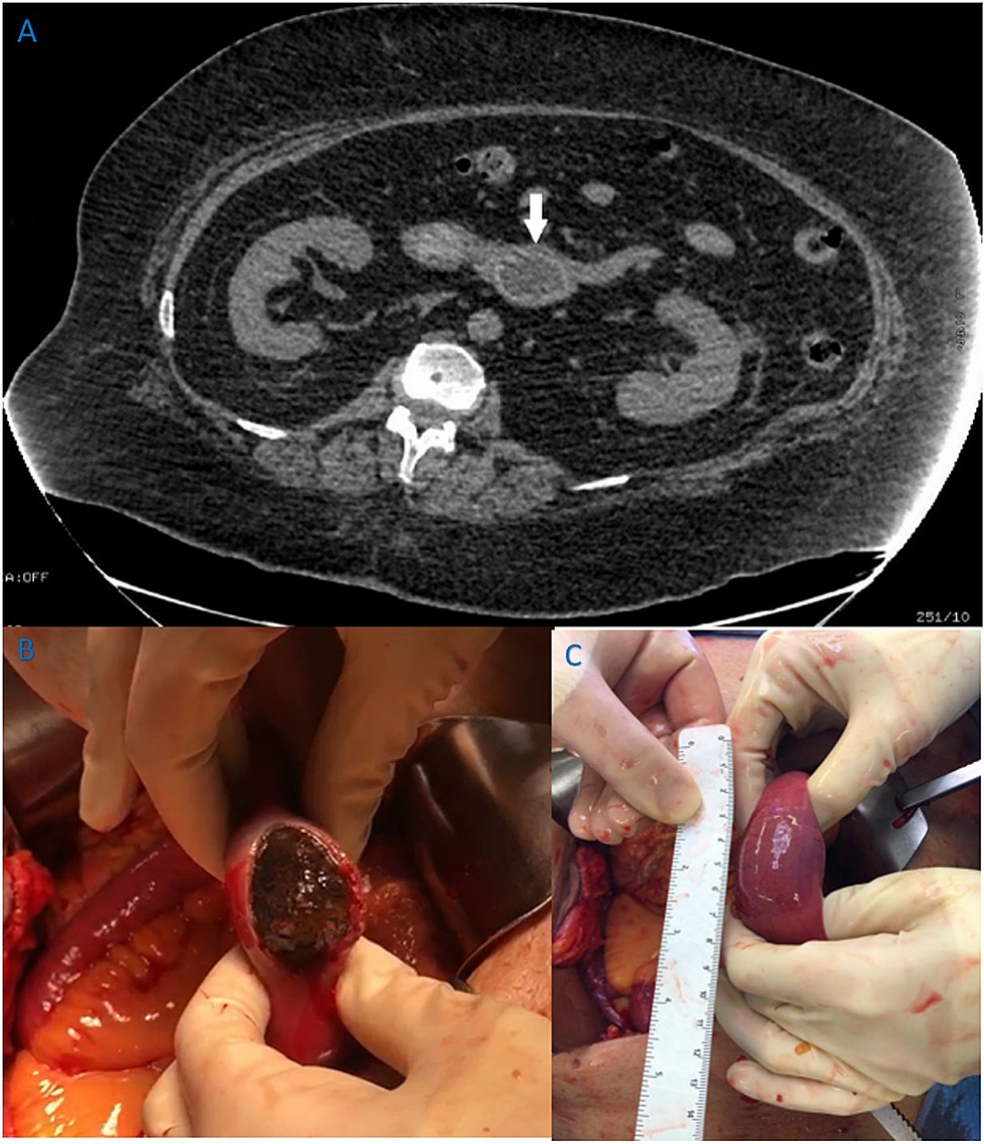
Gallstone ileus. (A) CT scan showing gallstone obstructing ileum (white arrow). (B) Obstructive gallstone in process of being gently removed from terminal ileum. (C) Impacted gallstone obstructing terminal ileum measuring roughly 5 cm × 3 cm × 4 cm found intraoperatively. CT: computed tomography.
